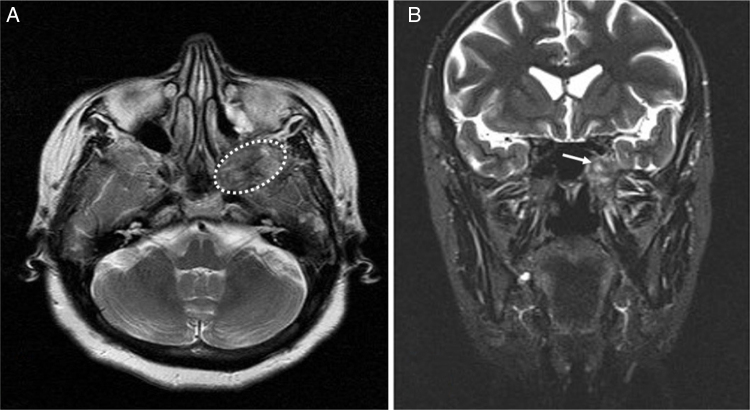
(A) Preoperative T1-weighted axial MRI. The mass is hypointense on the T1-weighted images (dashed-line indicates tumor). (B) Preoperative T2-weighted coronal MRI. The mass is mildly hyperintense on the T2-weighted images (arrow indicates tumor).
